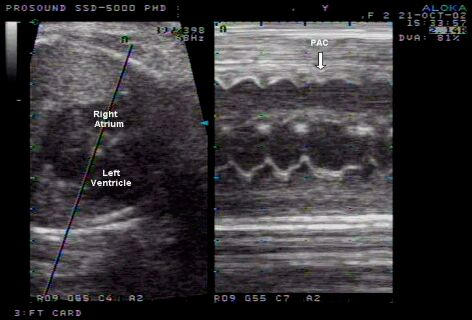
M-mode echocardiography of a premature atrial contraction (PAC)
